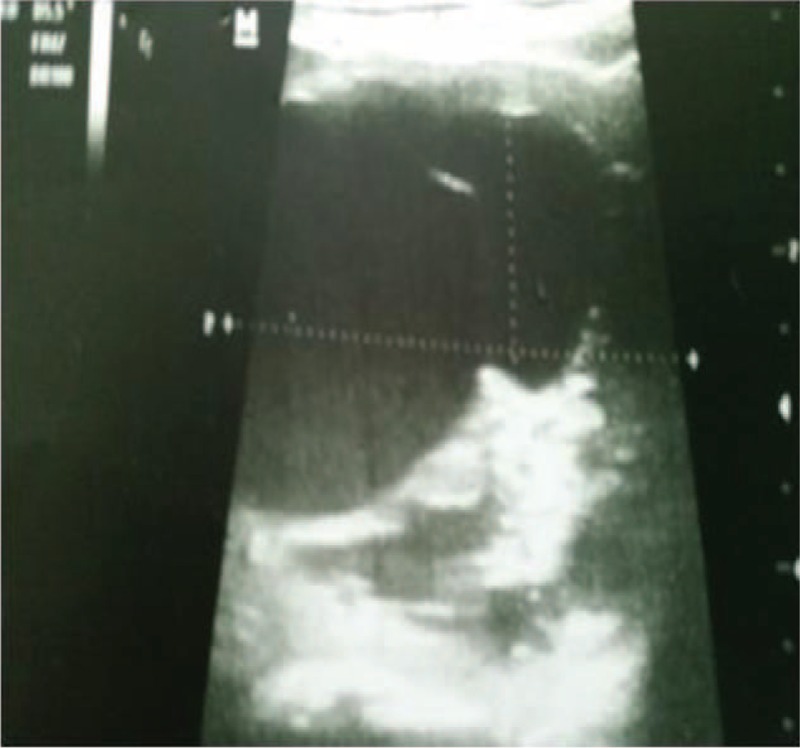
Ultrasonography of thyroid.
